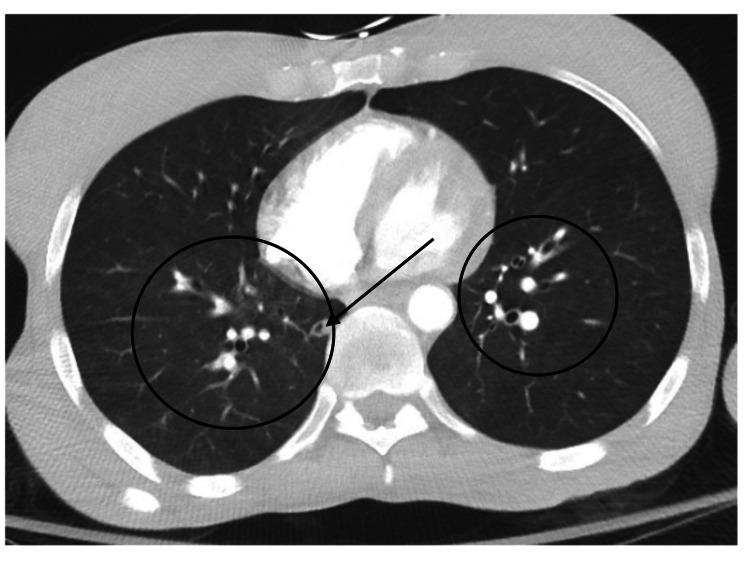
CT of chest at time of suspected IRIS, showing interval resolution of bilateral pulmonary infiltrate (circles) and stable cavitary lesion (arrow) IRIS: immune reconstitution inflammatory syndrome
